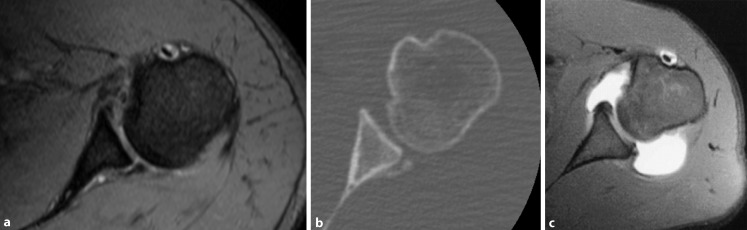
Structural dynamic posterior instability (B2). Dynamic structural posterior shoulder instability with posterior bony Bankart lesion visible on magnetic resonance (MR) (a) and computed tomography (b) images. MR arthrography of a patient with posterior capsular redundancy and posterior labral damage as an example of combined structural deficiency and structural damage (c)
